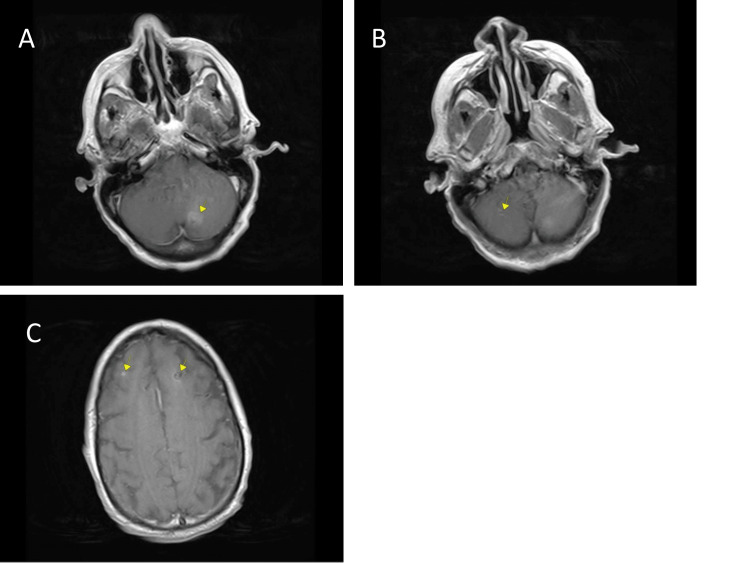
MRI Brain Axial T1 Post-Contrast A - 1.4 cm L cerebellar lesion; B - 3 mm R cerebellar lesion; C - 4 mm R anterior frontal lesion, and 1.1 cm L frontal lesion with hemorrhagic metastatic focus
